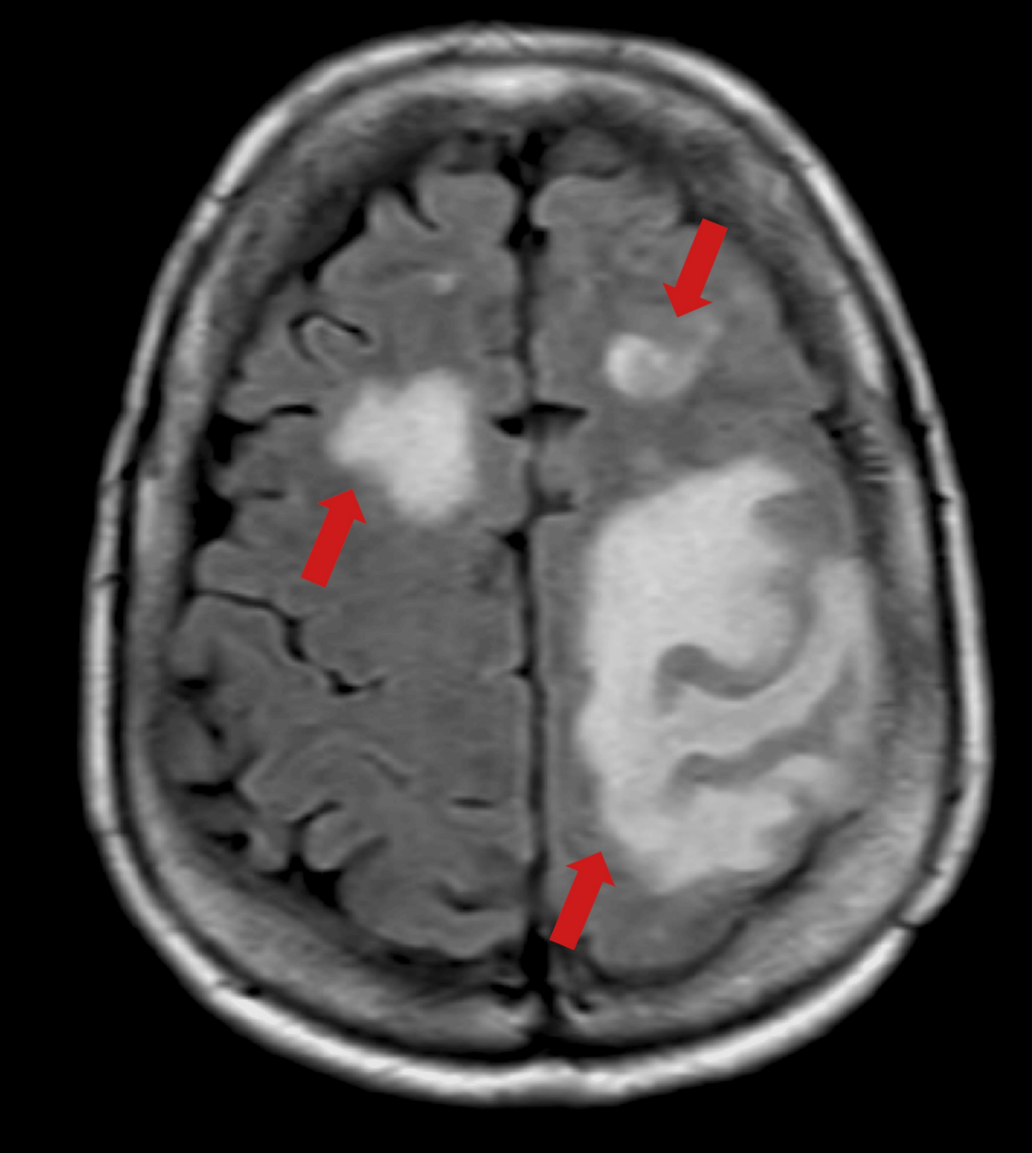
Brain MRI findings of intracranial IA-LPD Fluid-attenuated inversion recovery (FLAIR) MRI reveals patchy hyperintense signals involving the right frontal and left temporal lobes, extending toward the frontal and parietal regions. IA-LPD: immunodeficiency‑associated lymphoproliferative disorder.
